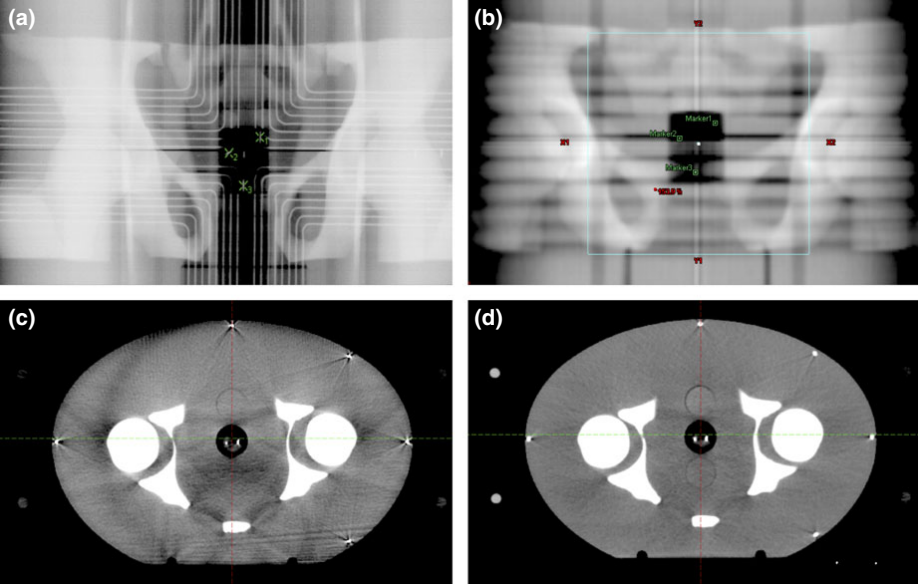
(a) Anterior kV image showing the Calypso transponders (green crosses) and copper wires in electromagnetic array, (b) planning DRR with markers indicating Calypso transponders, (c) CBCT, and (d) planning CT scan.
